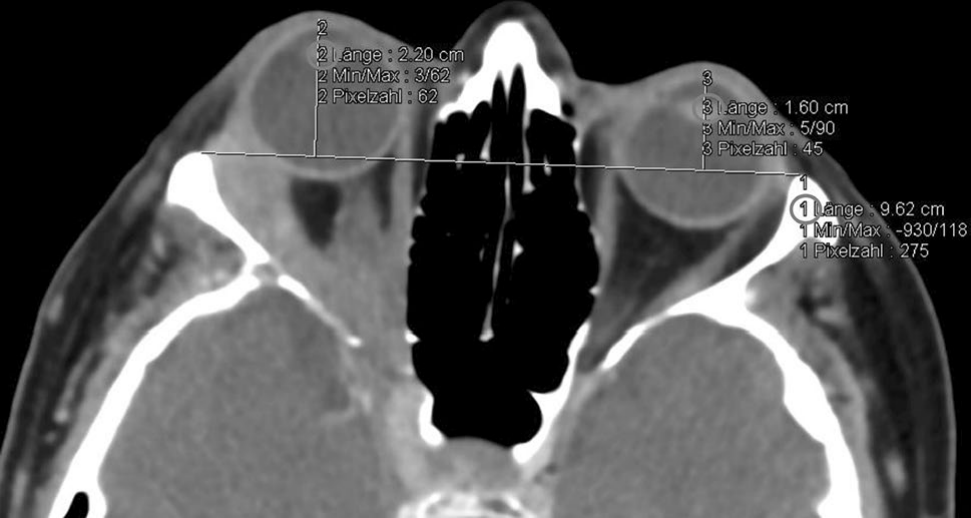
Exemplary CT measurement in the axial plane bisecting the lens: Distance between the lateral orbital rims (1) and perpendicular distance to the corneal apex (2) and (3) in a patient with 6 mm proptosis of the right eye due to adenoidcystic carcinoma of the lacrimal gland with deep orbital invasion (CT with contrast agent, soft tissue window).
